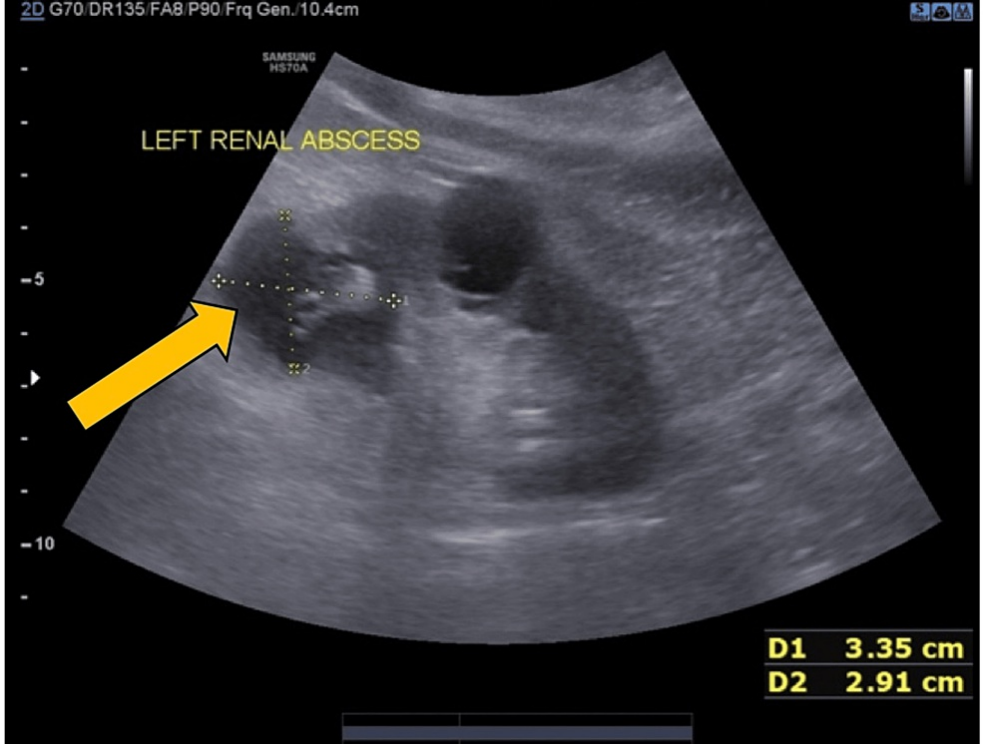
Ultrasound of the abdomen revealing a well-defined hypoechoic cystic lesion with a thin wall with debris and multiple echoes noted at the upper pole of the right kidney
